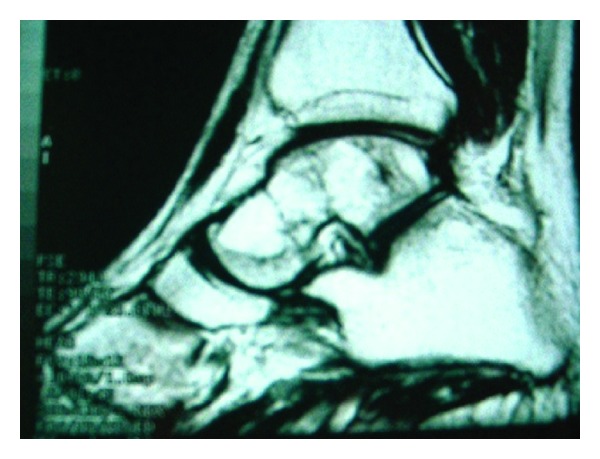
Fat containing cystic lesions surrounded by smooth, thick rims with septations in both talus have been shown on MRI.
